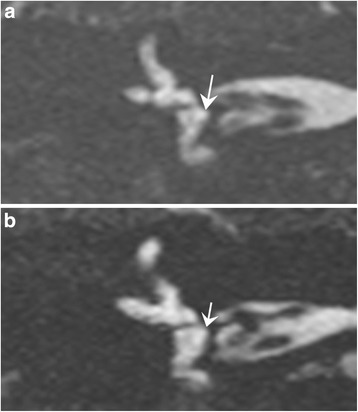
3T MRI. Coronal T2-weighted gradient-echo FIESTA-C images in two patients suffering from Meniere disease. 5 a) Dilated saccule with an increased saccular height (> 1.6 mm), associated with an increased saccular width (> 1.4 mm). 5 b) Round saccule with an isolated increased saccular width > 1.4 mm
